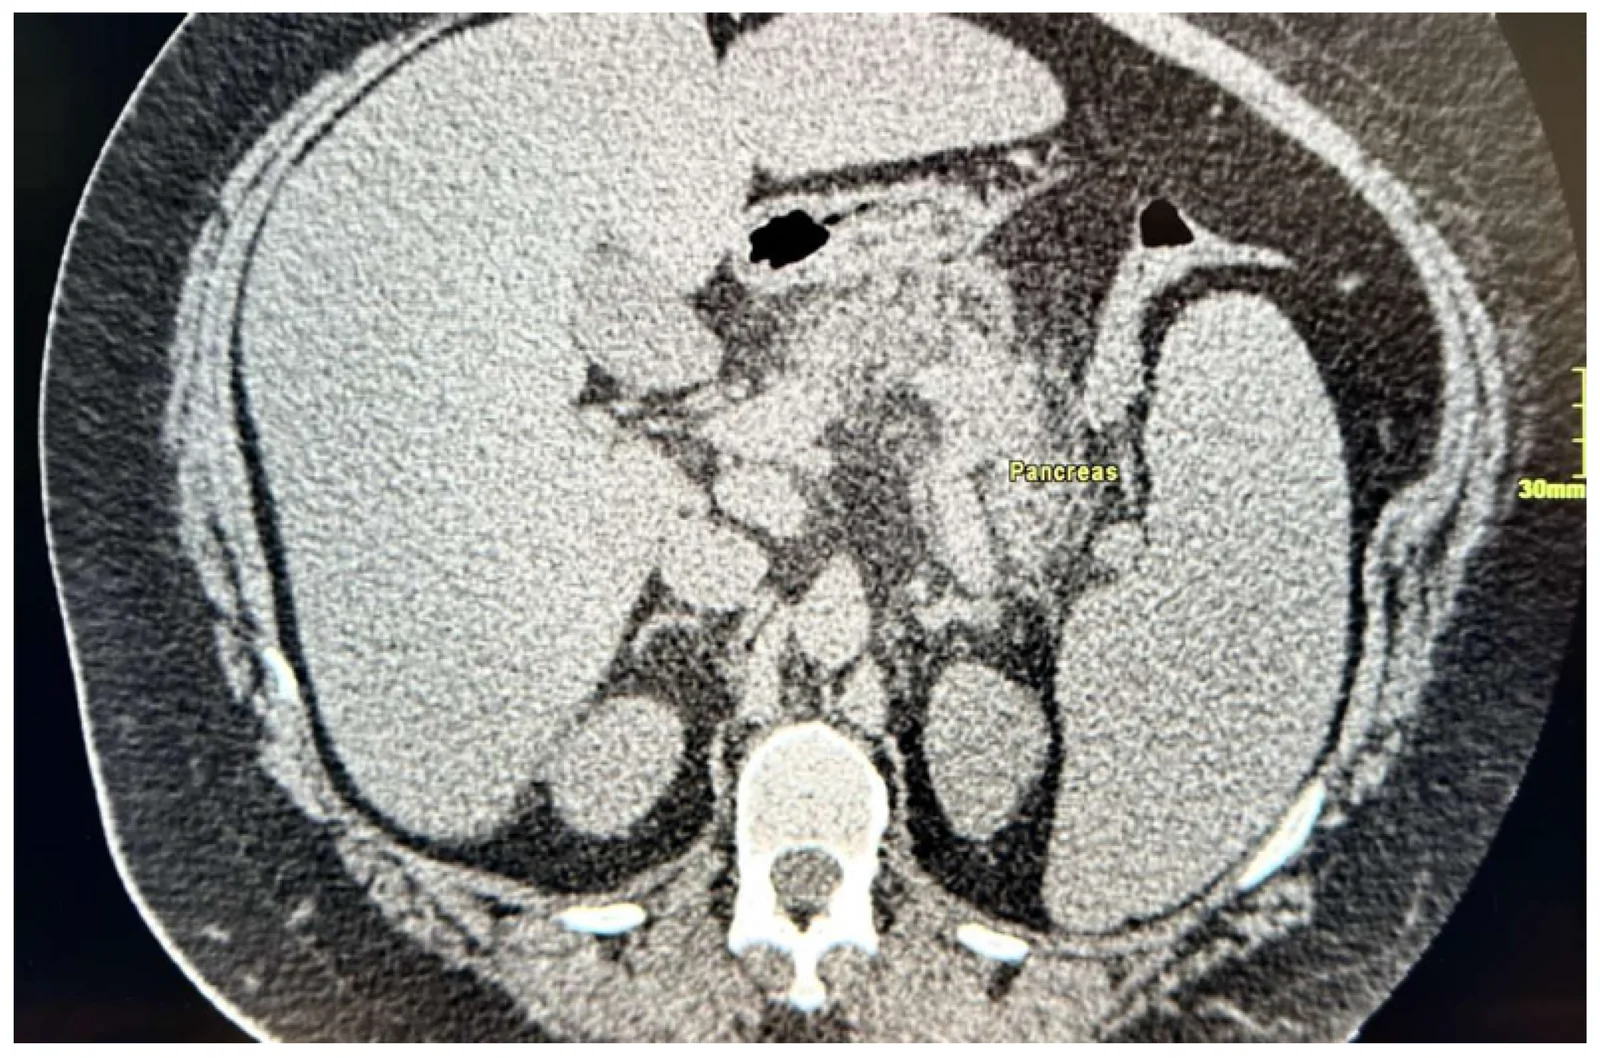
CT abdomen showing hepatosplenomegaly, lymphadenopathy and peripancreatic oedema and free fluid consistent with acute pancreatitis.
